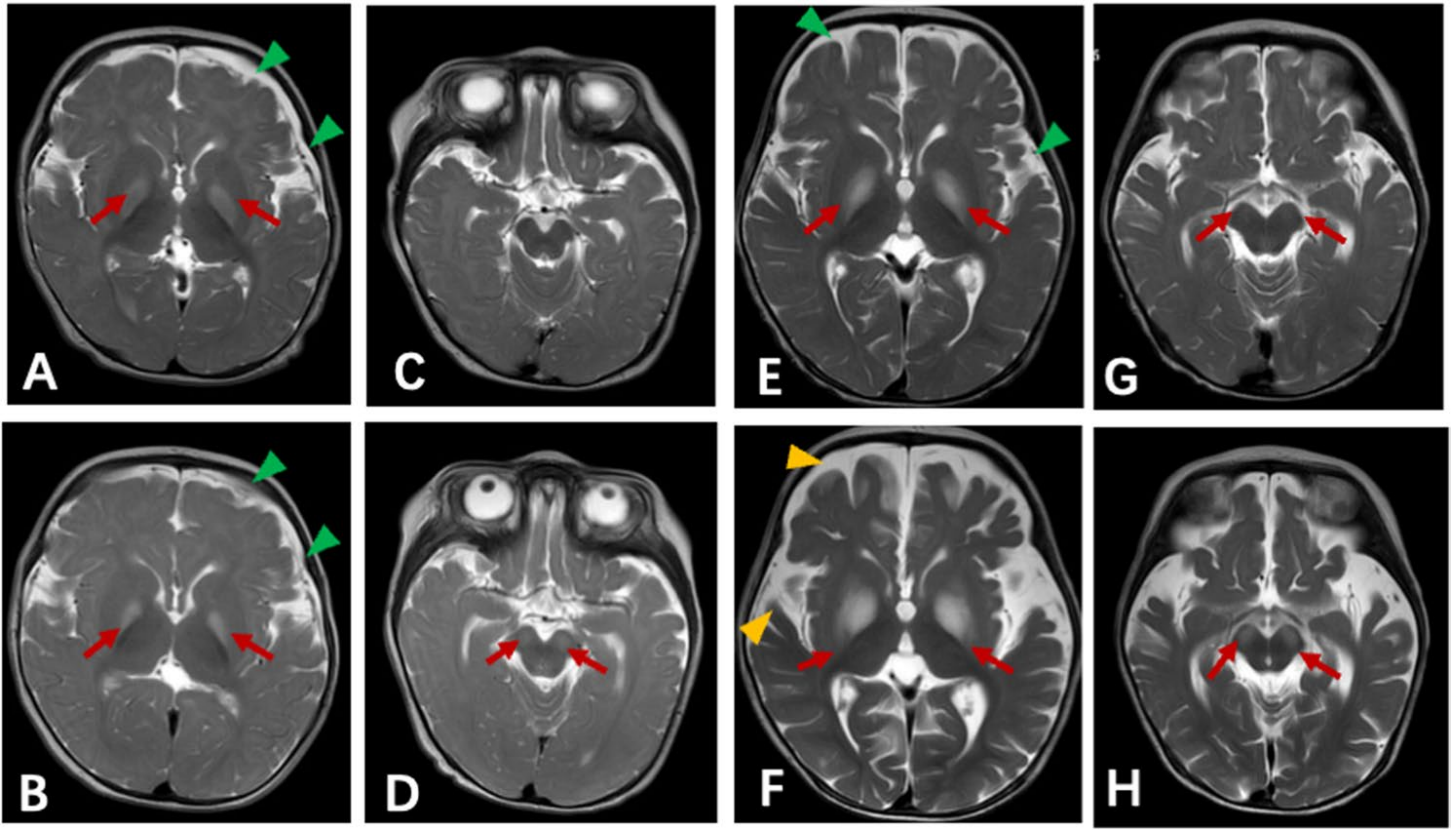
Brain MRI findings in two patients with mild *MOCS2*-related MoCD-B. Axial T2-weighted images of Patient 1 (A–D) and Patient 2 (E–H). Top panels (A, C, E, G) were obtained at disease onset, and bottom panels (B, D, F, H) on repeat MRI during the same hospitalization. Red arrows indicate symmetric hyperintensities in the bilateral globi pallidi and cerebral peduncles. Green and yellow arrowheads indicate enlarged extracerebral spaces
